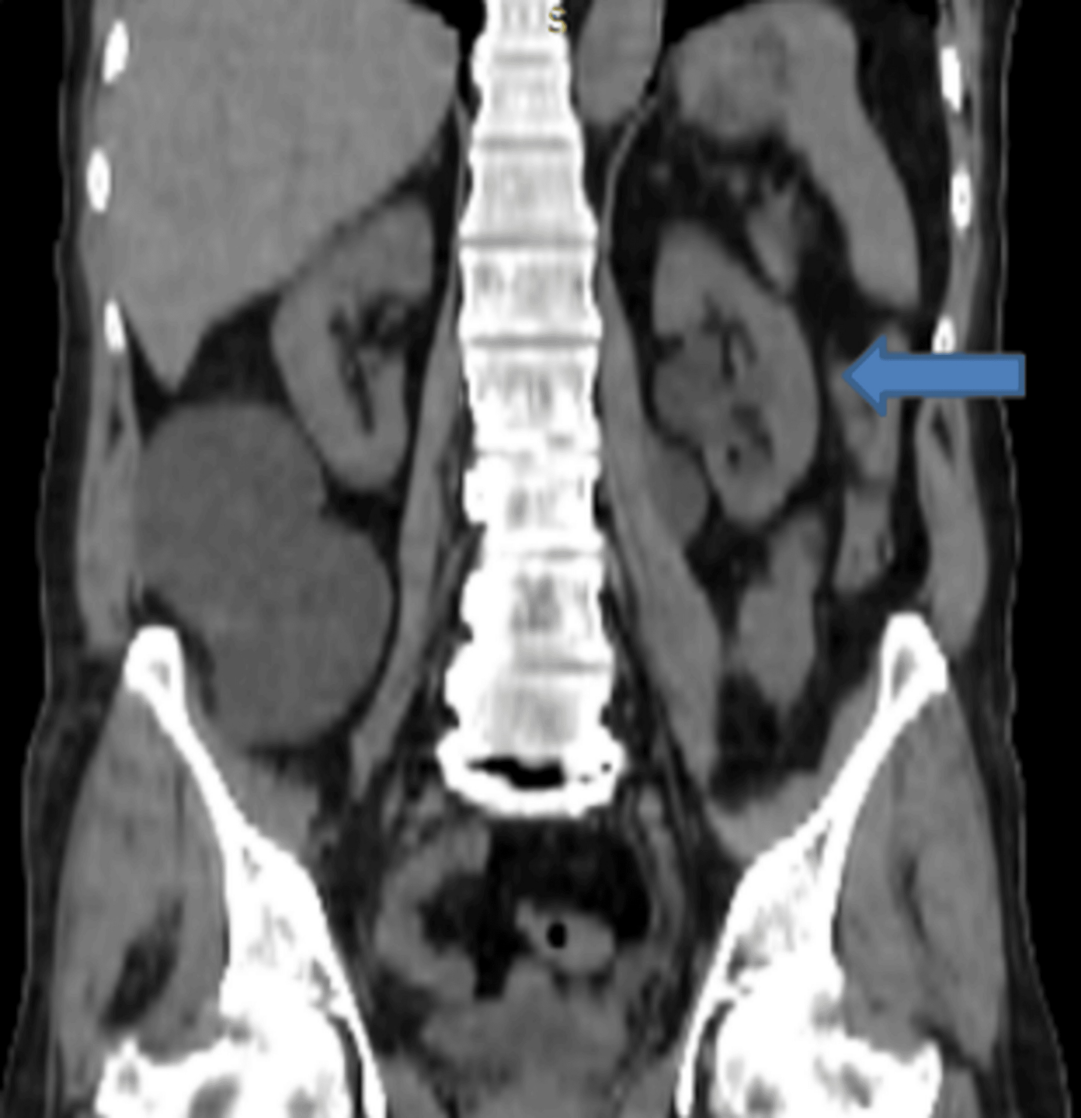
Non-contrast CT of the abdomen (coronal view) showing mild dilatation of the left renal collecting system (blue arrow).
